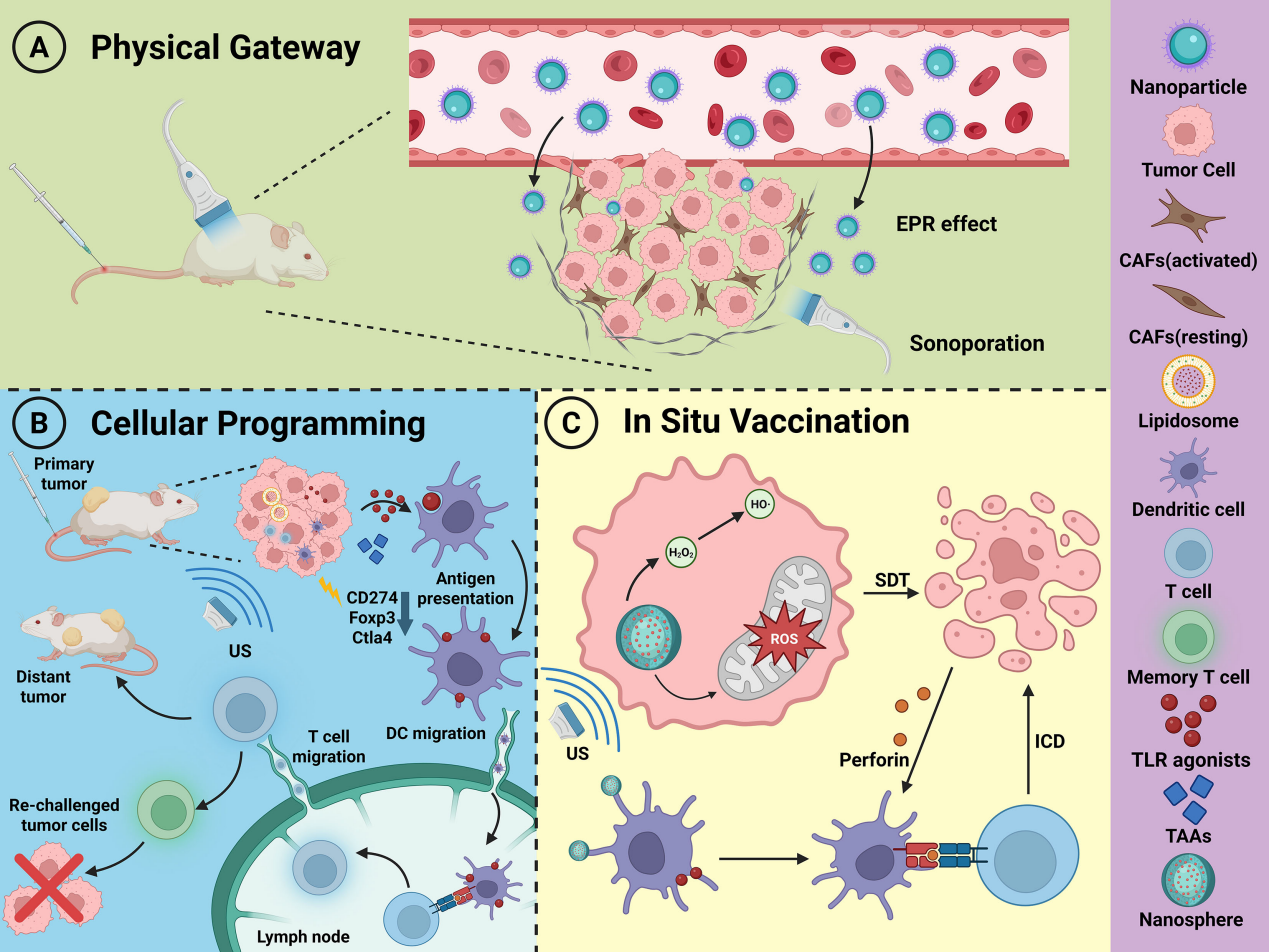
Multifunctional roles of ultrasound in acoustic immune reprogramming (Created in https://BioRender.com): (A) Physical Gateway: Ultrasound, in combination with microbubbles, temporarily and reversibly opens biological barriers (e.g., vascular endothelium, BBB) through sonoporation, enhancing the infiltration of immune cells and therapeutic agents (61). (B) Cellular Programming: Low-intensity focused ultrasound (LIFU) can precisely modulate specific cell functions, such as reprogramming neuronal activity to regulate systemic immunity via the neuro-immune axis (44, 48) or directly enhancing T cell activation through mechanostimulation (62). (C)*In Situ* Vaccination: High-intensity ultrasound ablation (e.g., HIFU) induces ICD, releasing tumor antigens and danger signals to initiate a systemic anti-tumor immune response akin to an endogenous vaccine (63).
